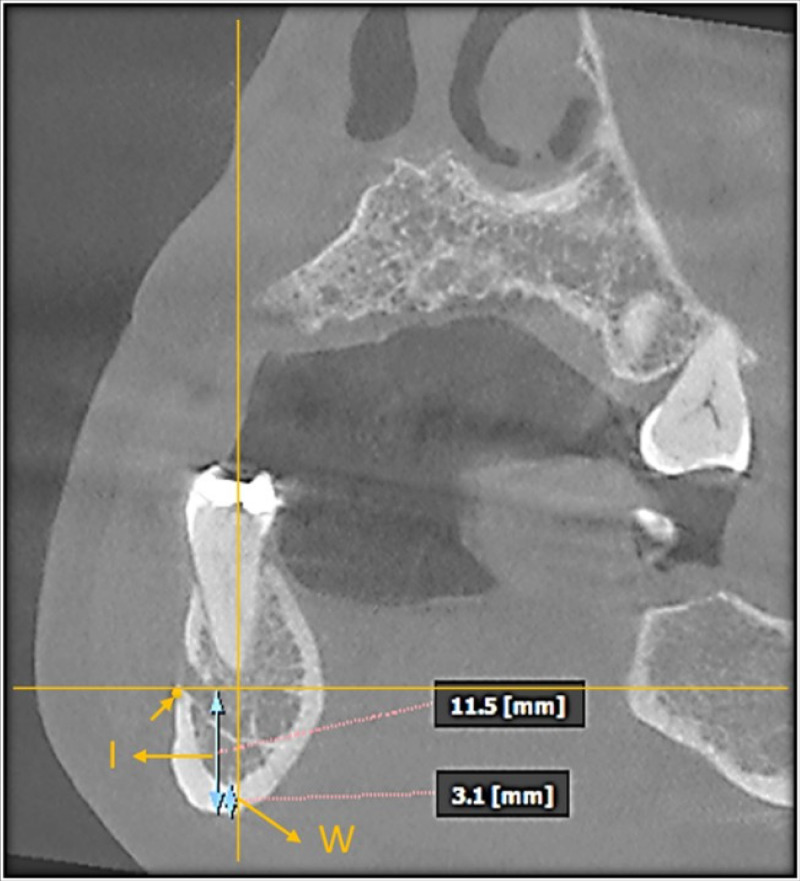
CTI(I) - computed tomography mandibular index.
